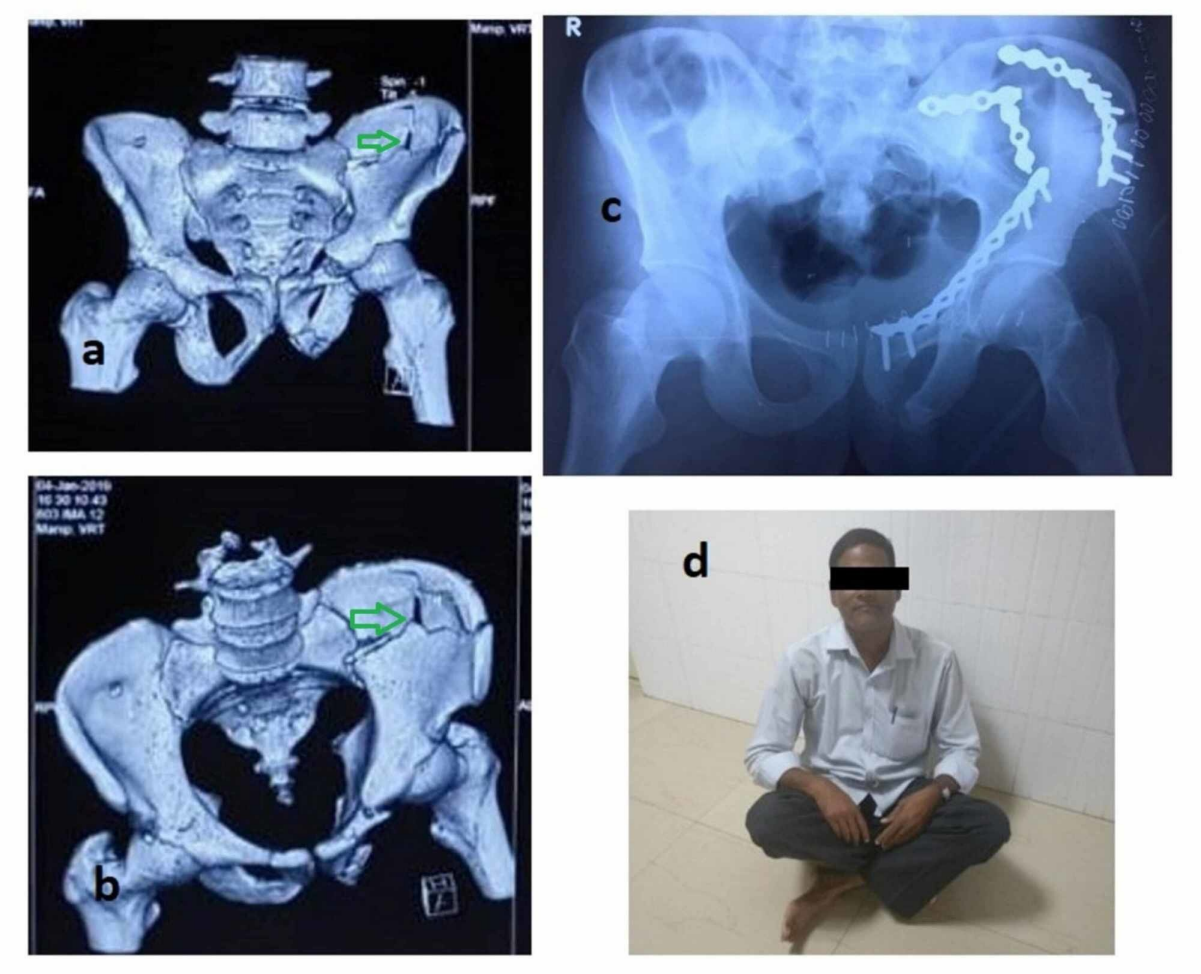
A case of anterior column fracture extending high in iliac blade (green arrows) with final postoperative X-rays and functional outcome a, b: preoperative CT of the pelvis with both hips; c: follow-up radiograph; d: follow-up clinical photograph of the patient sitting cross-legged CT: computed tomography
